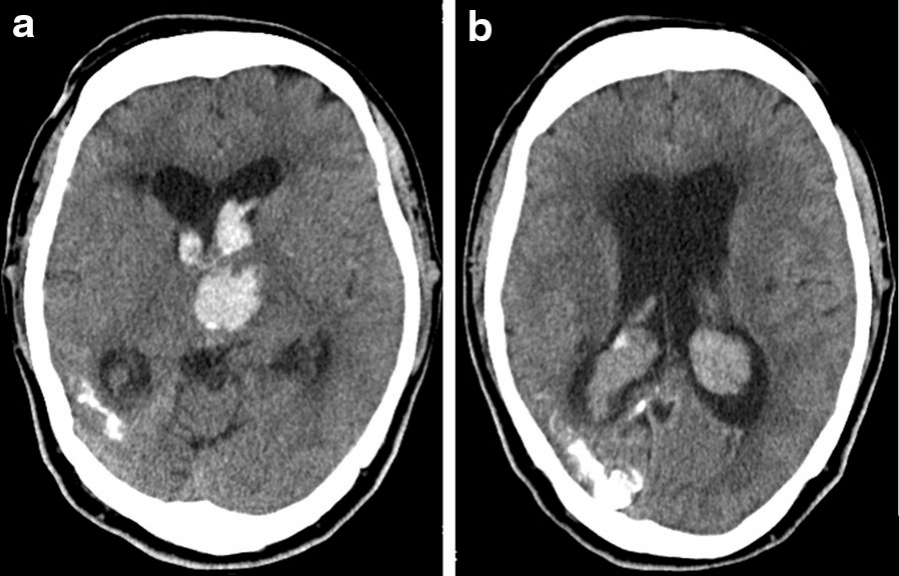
a CT on admission showing left thalamic hemorrhage and ventricular hemorrhage with acute hydrocephalus. b Bilateral choroid plexus enlargement and subcortical calcification were detected in the right temporo-occipital lobe
